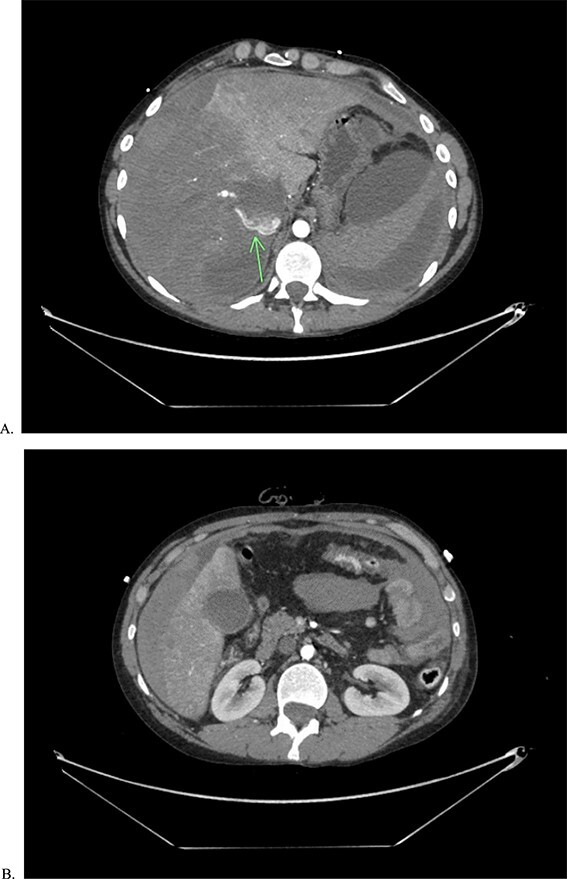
CTA of abdomen and pelvis showing the large contrast extravasation (A) as well as significant amount of free fluid in the abdomen (B).
